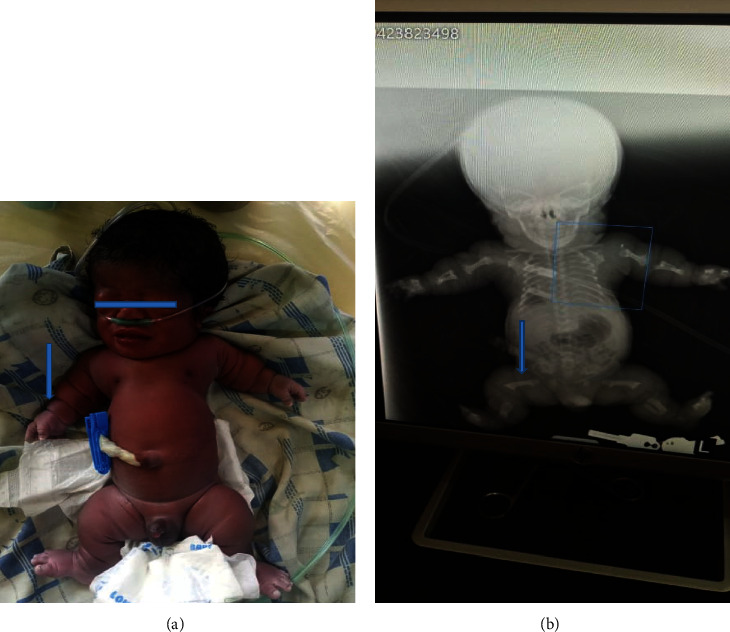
(a) A clinical photograph of baby 2 with thanatophoric dysplasia: large head prominent skin folds (arrow), narrow chest, and short limbs. (b) A plain radiograph of baby 2 with features of TD type 1. The arrow shows a curved telephone-handle femur.
